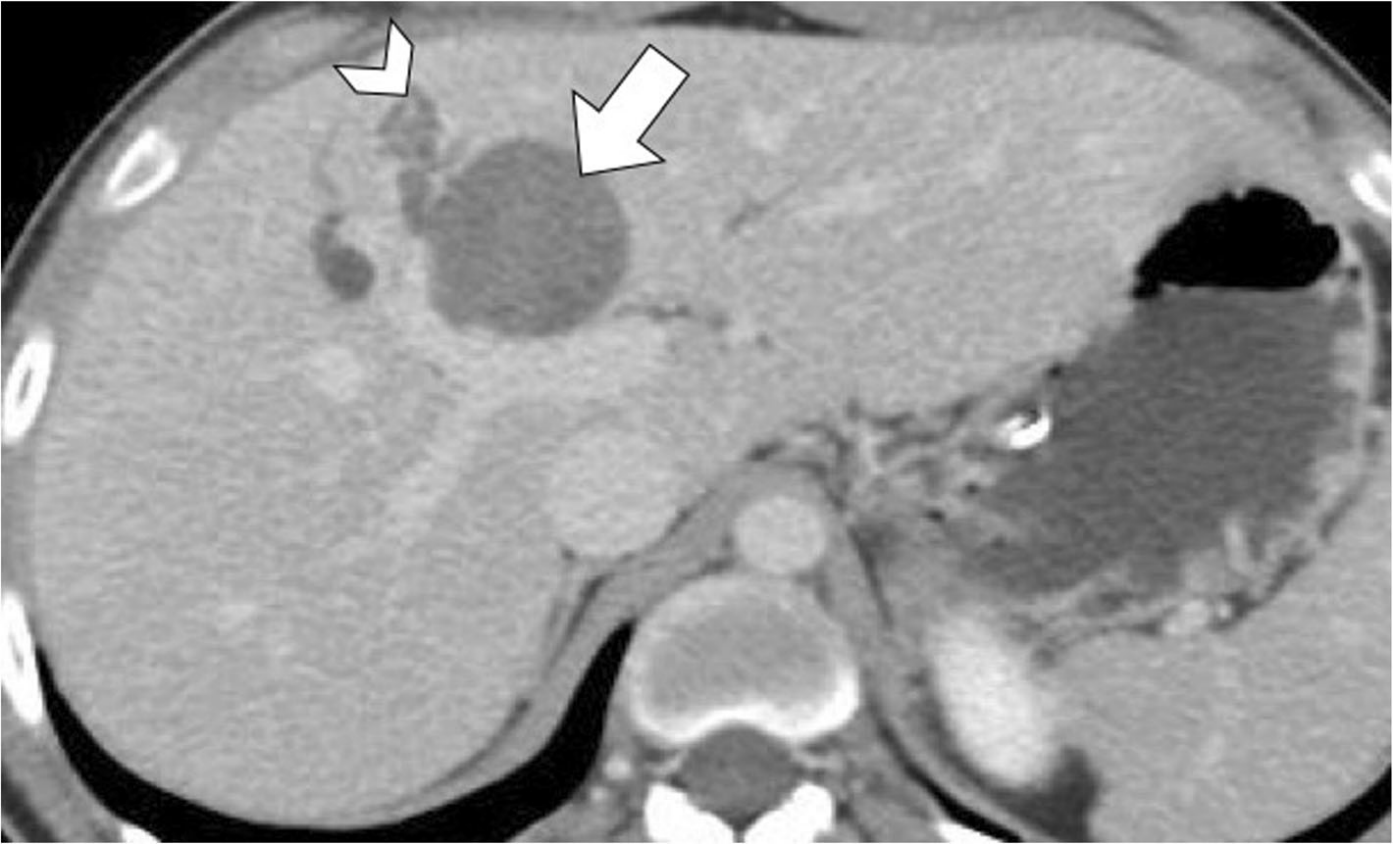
A 21-year-old man with hepatic hydatid cyst. Axial contrast-enhanced CT image on portal venous phase shows hepatic hydatid cyst (arrow) causing compression and dilatation of adjacent intrahepatic bile ducts (arrowhead)
